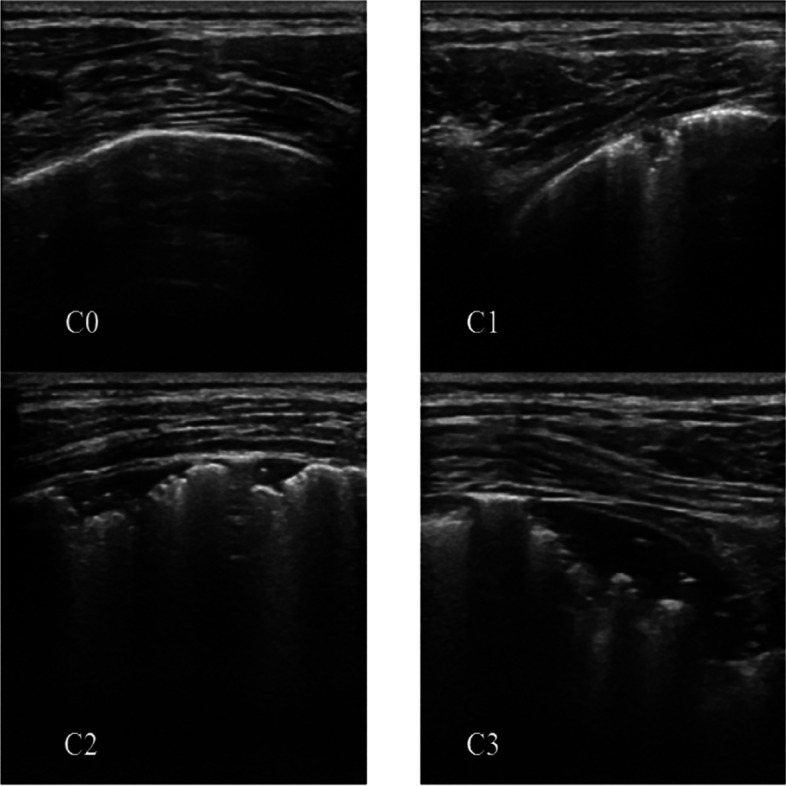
Ultrasound images of pulmonary atelectasis and grading of atelectasis
